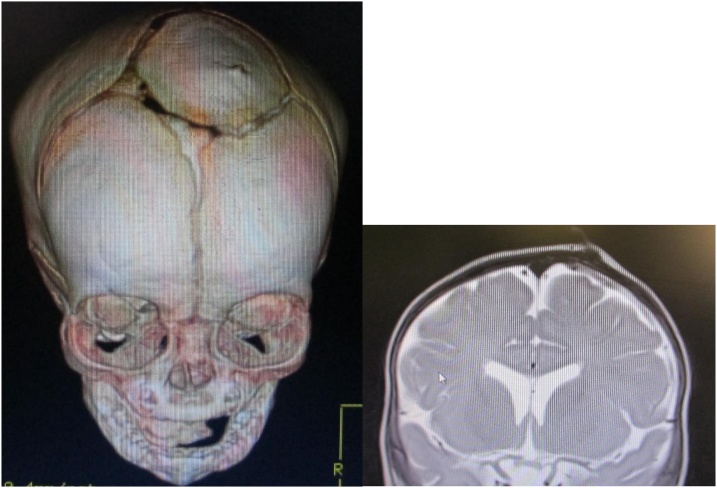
3D computed tomography and magnetic resonance imaging showing abnormal bony island and connecting vessel. MRI showed a soft tissue skin tag in the upper left frontal parasagittal region, with a tiny vascular connection to the intracranial cortical vein over the brain surface 2–3 mm from the sagittal sinus. (On the left, 3D-CT of skull shown a small central bony spur corresponding to the skin tag area. On the right, MRI showed a soft tissue skin tag in the upper left frontal parasagittal region, with a tiny vascular connection to the intracranial cortical vein over the brain surface 2–3 mm from the sagittal sinus).
